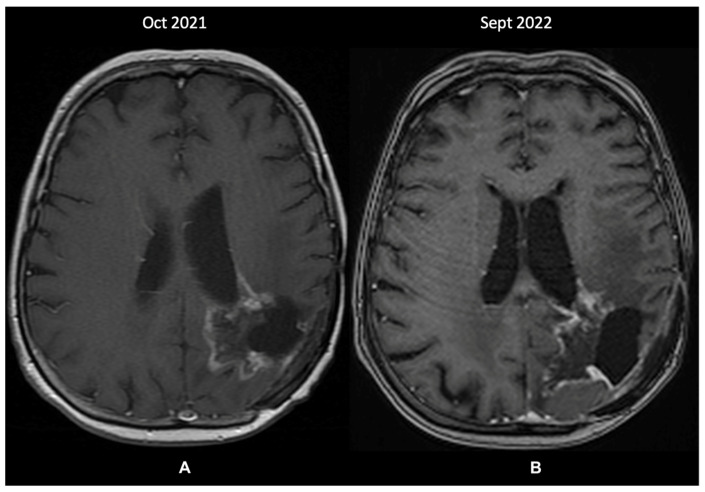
The patient’s last 2 MRI scans, T1 + contrast sequence (October 2021 and September 2022): (A)—changes within the left parietal lobe suggestive of recurrence and radiation-induced necrosis, extending medially from the postoperative cavity, around the subependymal space adjacent to the posterior horn of the left ventricle; (B)—discrete changes towards a probable tumor recurrence in the posterior part of the cystic cavity.
